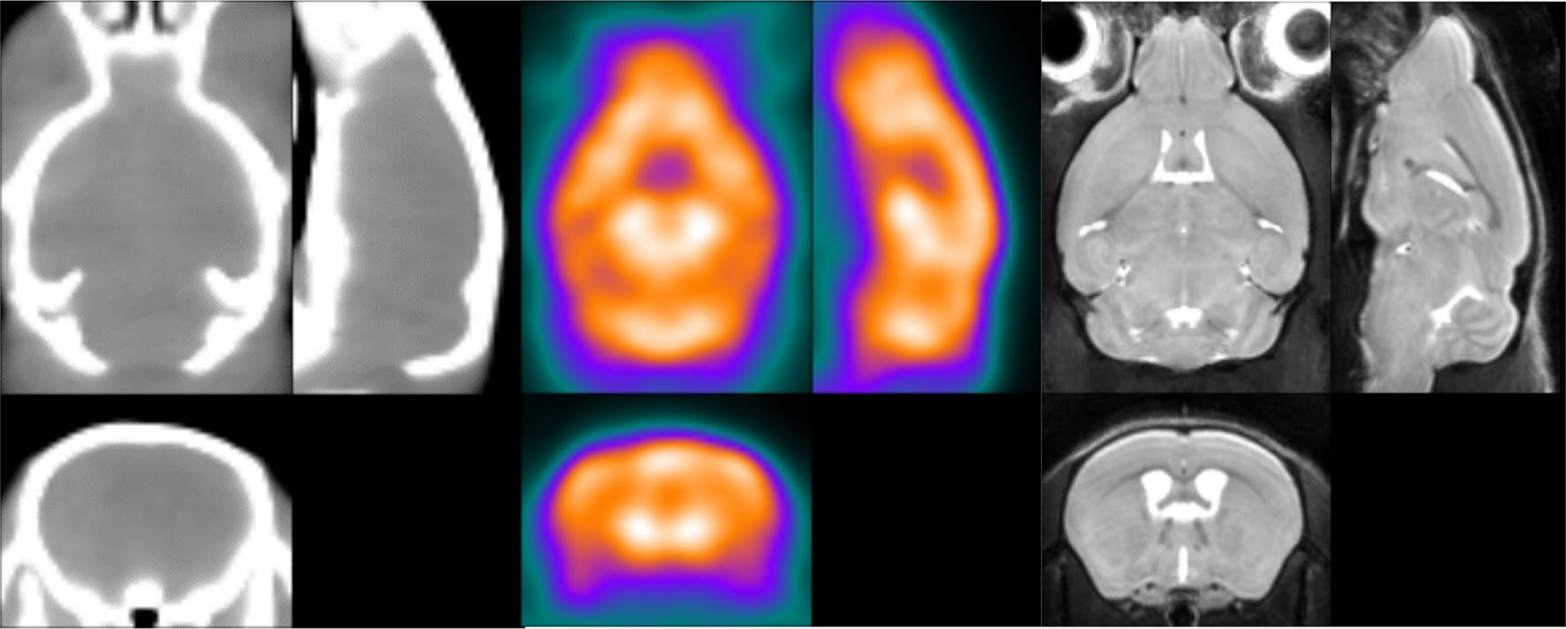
Three-plane view of the CT (right), PET (center) and MR (right) image templates obtained with the proposed normalization method, aligned to the ARA.
